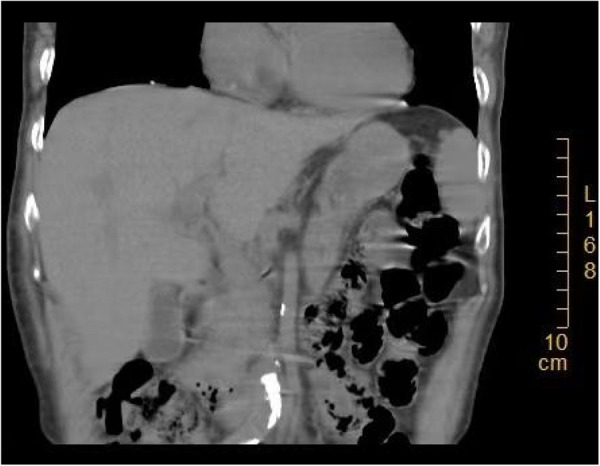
The upper abdominal CT scan revealed a postprandial gallbladder with a slightly thickened and coarse gallbladder wall.
